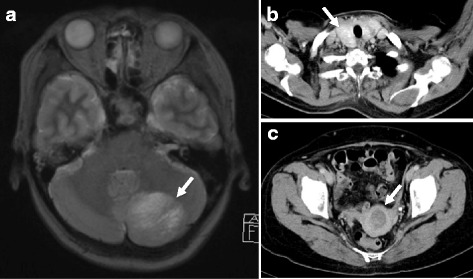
MRI and CT at the age of 50 years. a MRI showed alternating isointense and hyperintense bands in the left cerebellar hemisphere (*arrow*). b Whole-body CT screening detected an adenomatous goiter (*arrow*). c CT also demonstrated uterine cancer (*arrow*)
